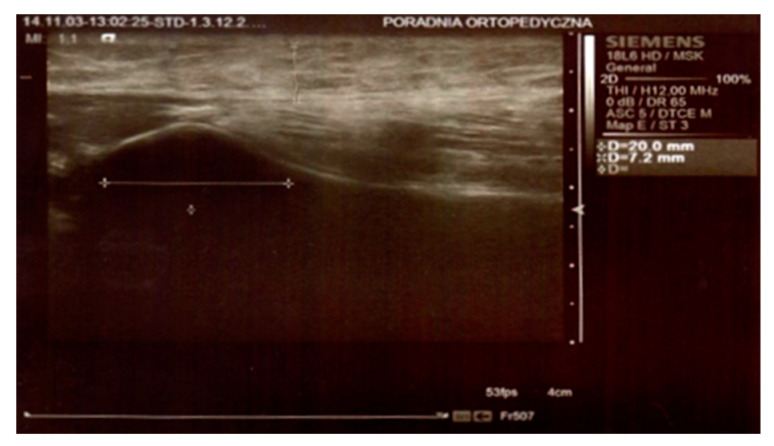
Ultrasound scan. Measurements at the top of the head of the fibula.
